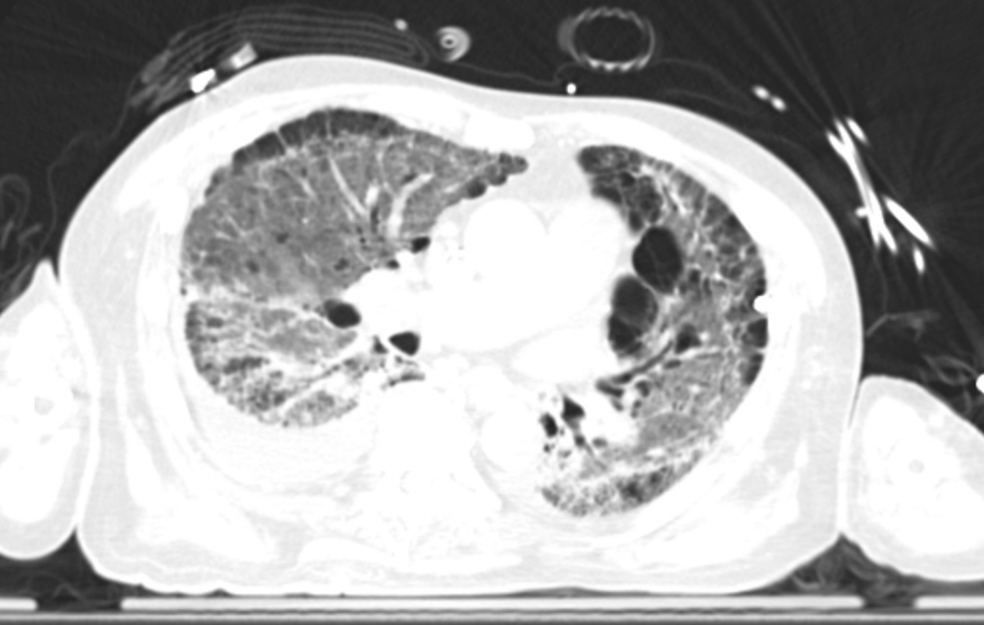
Repeat chest CT scan showing the progression of ground-glass opacities and reticulation in bilateral upper lobes and lower lobes, with worsening diffuse bilateral subpleural reticular opacities with the suggestion of honeycombing
